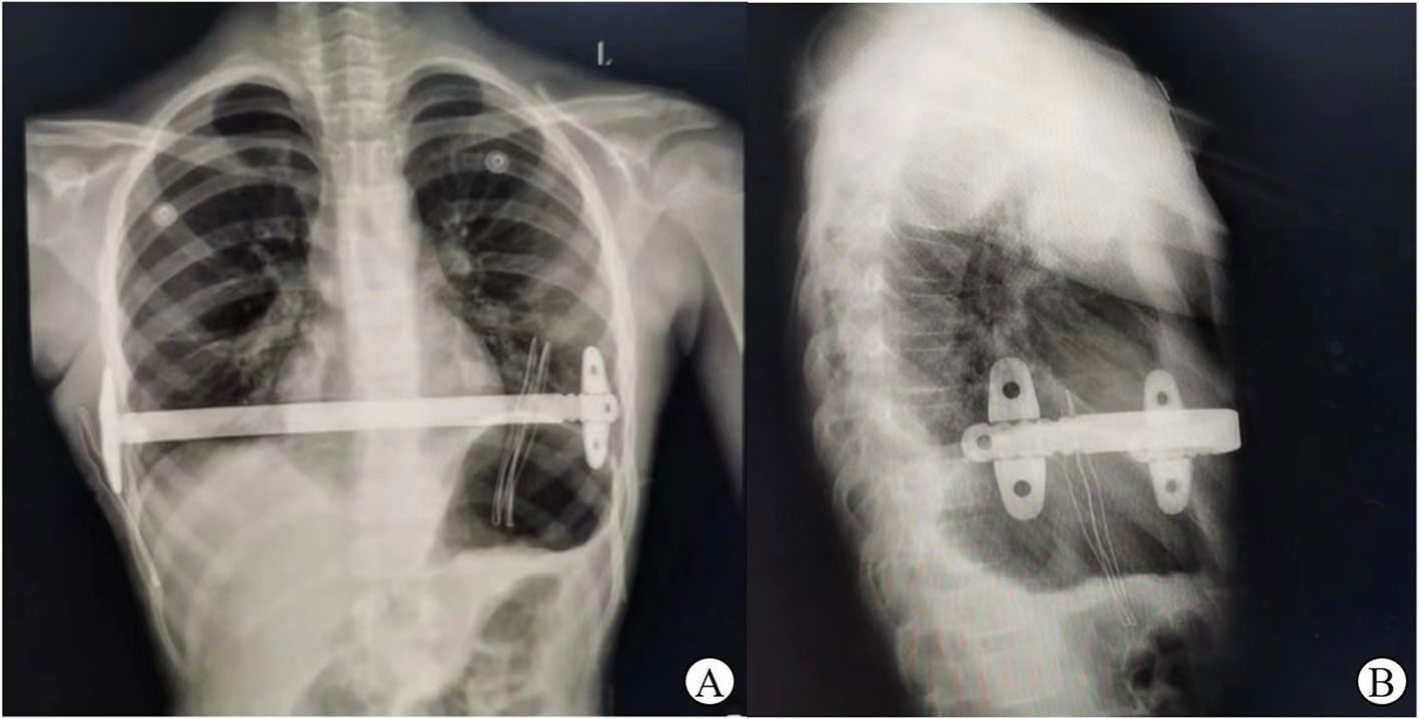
Bar position at postoperative chest X-ray: posteroanterior (A) and laterolateral (B) views
